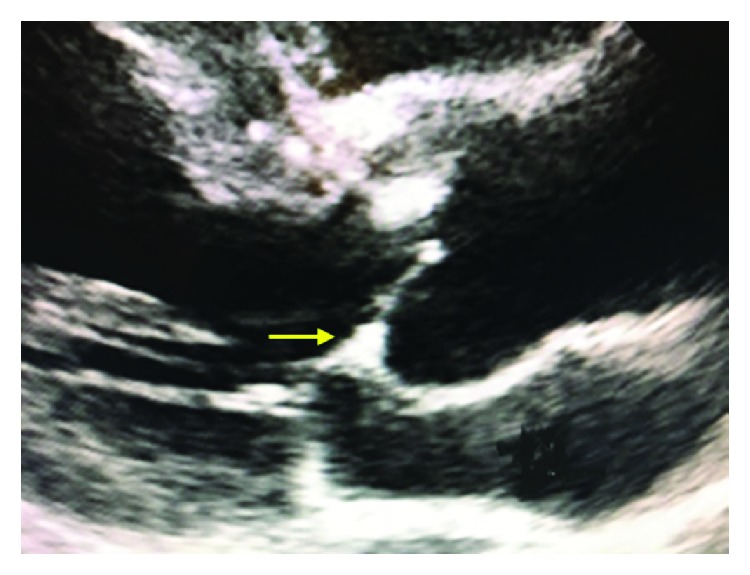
Preoperative transthoracic echocardiography. Apart from a slightly thickened aortic valve (arrow), no clear abnormalities indicative for a hemangioma could be observed.
